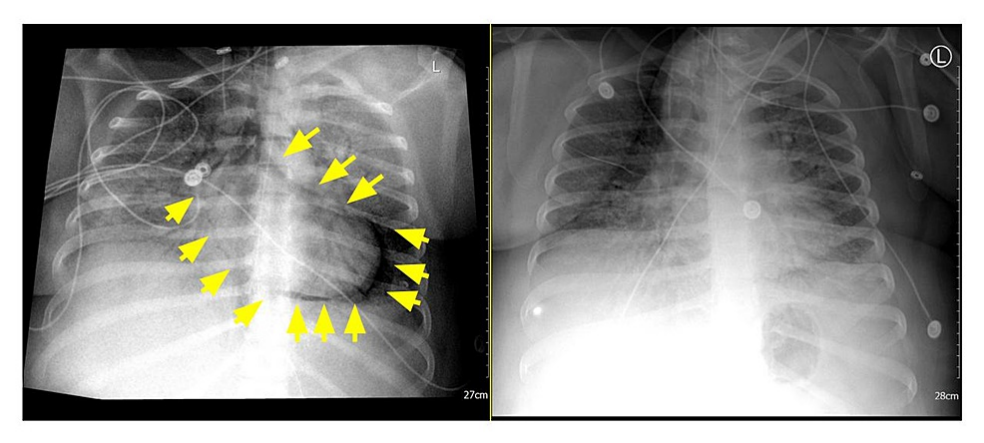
Chest X-ray 24 hours before and after pneumopericardium. Left: Chest X-ray shows ill-defined opacities and multifocal opacities. The enlarged cardiac silhouette is also present and may represent CHF pattern (yellow arrows: pneumopericardium). Right: X-ray shows linear lucency surrounding the cardiac shadow representing pneumopericardium.
